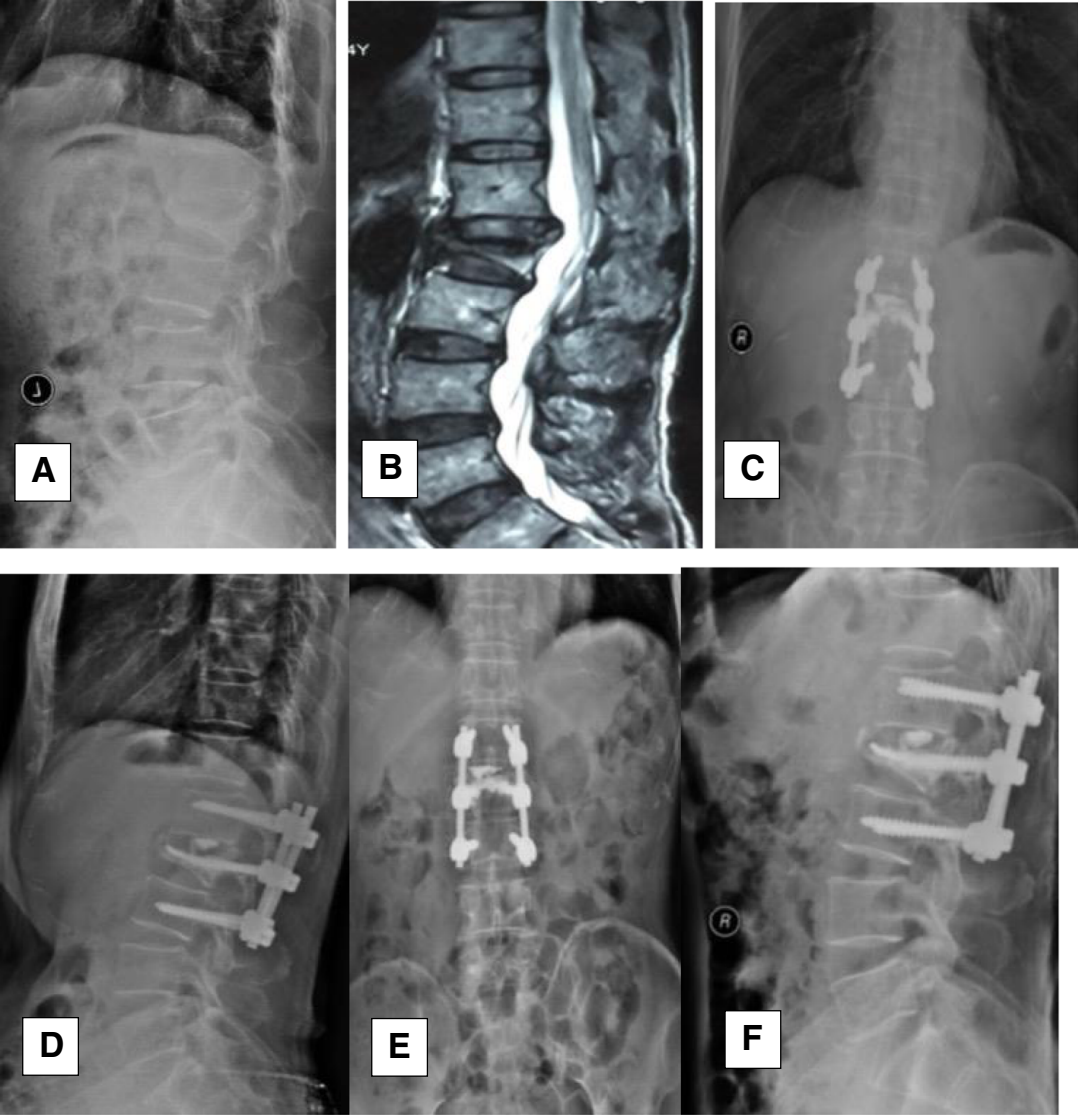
A 70-year-old male patient with an L2 osteoporotic fracture was treated surgically with PV combined with IBPSF. ab The first admission X-ray and MRI showed a lumbar compression fracture at L2, and he underwent surgical treatment. cdef At the 1-month follow-up and final follow-up, X-ray showed that the fractured vertebral height had been restored, kyphosis was sufficiently corrected, and low levels of cement leakage were observed
